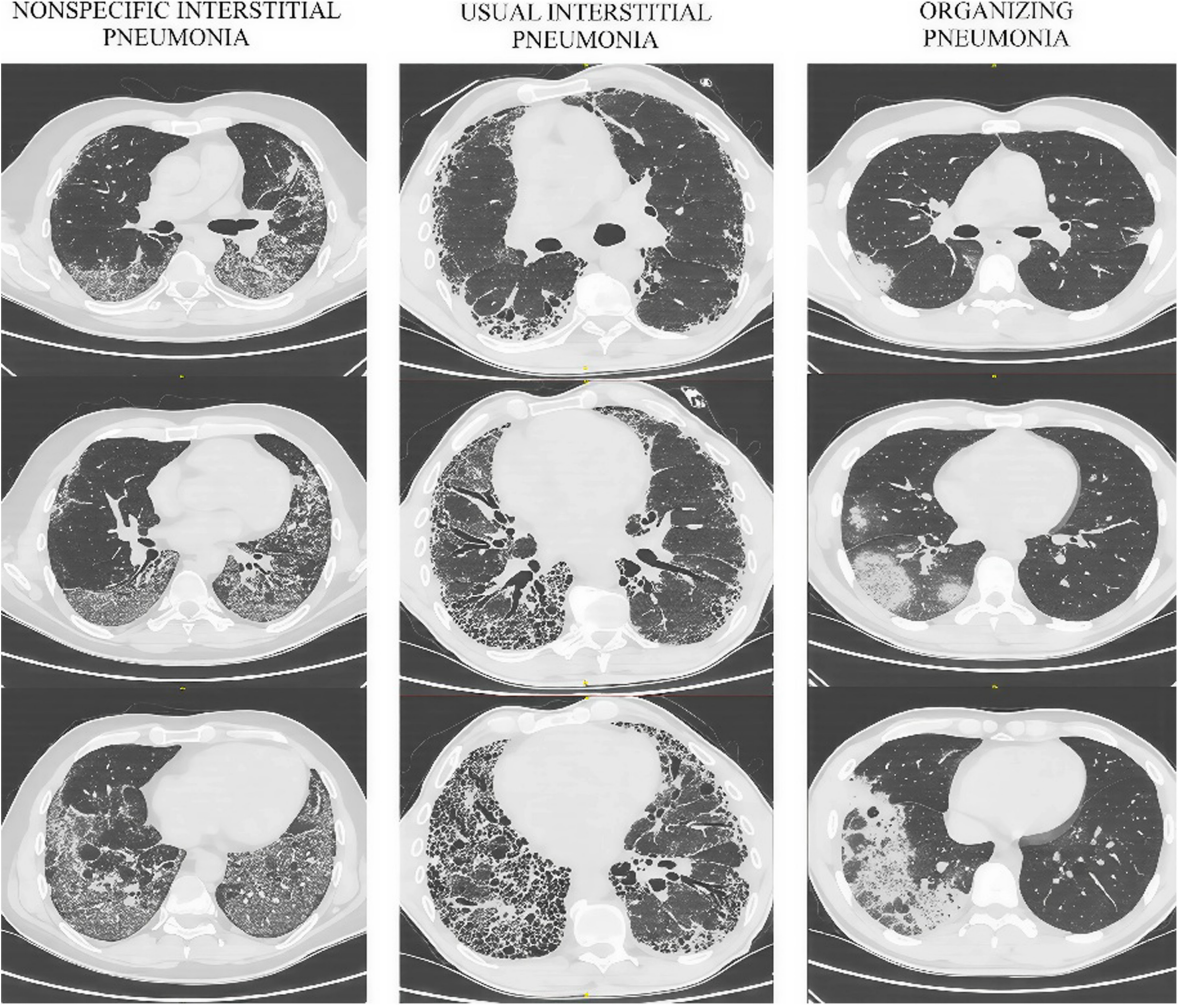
Common radiographic signs and patterns in interstitial lung disease visualized in HRCT.
